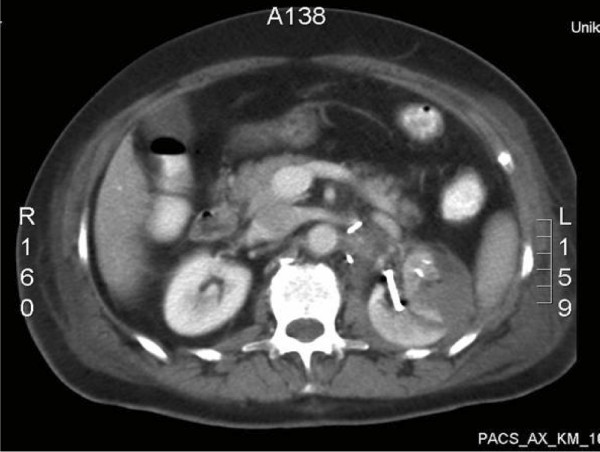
CT scan of a patient with bleeding from the tumor bed. The SIS secured the defect and stopped the bleeding by compressing the defect.
